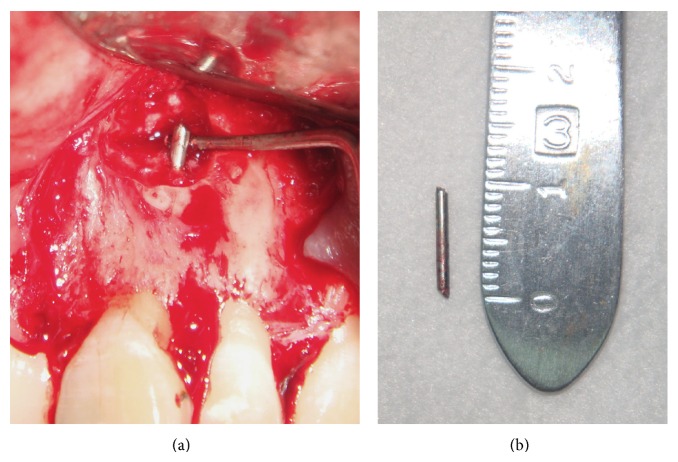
(a) The clinical appearance of the metal fragment located in the periapical tissues. (b) An image showing the length (approximately 10 mm) of the metal foreign body that was removed during the surgical procedure.
